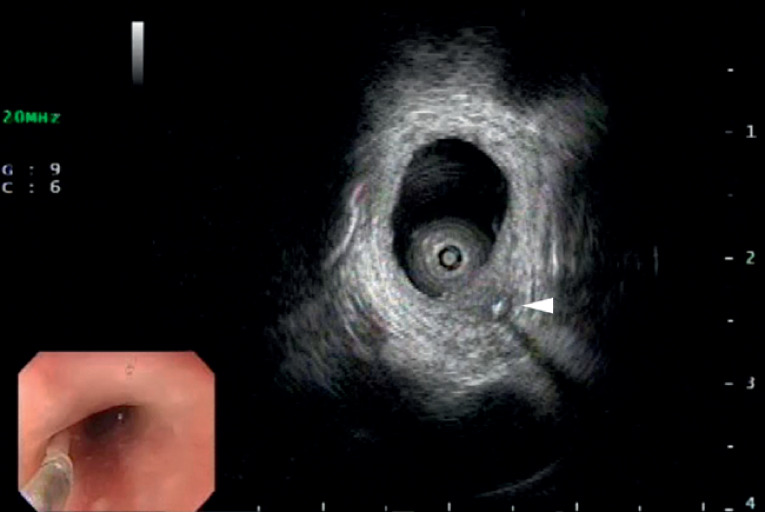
Endoscopic ultrasound image using a miniature probe with a novel gel immersion technique clearly showing the whole embedded fishbone beneath the mucosa.
